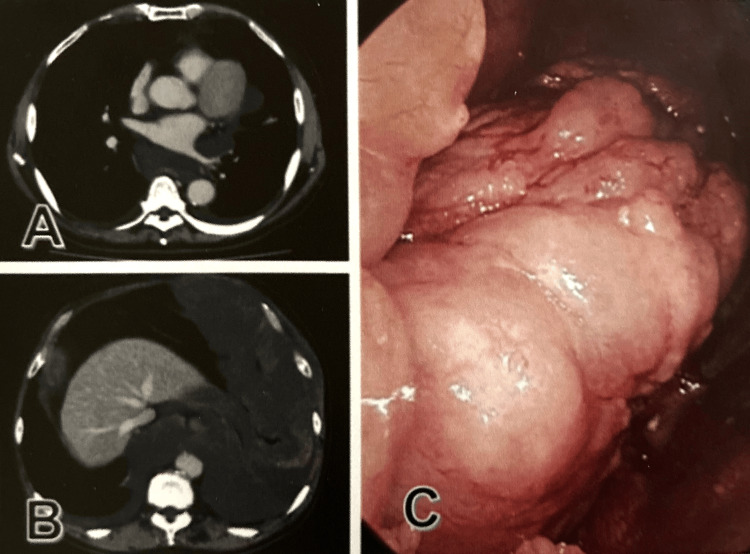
CT and endoscopic findings A-C: A multinodular cystic lesion widely developing on the pleura from the mediastinum to the diaphragm (A, B: CT; C: endoscopy). CT: computed tomography
